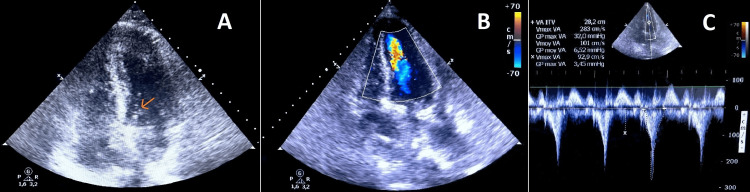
Immediate postoperative transthoracic echocardiogram A: Mitral valve systolic anterior motion (arrow). B: Intraventricular aliasing. C: Dagger-shaped spectral Doppler with a peak gradient of 32 mmHg.
